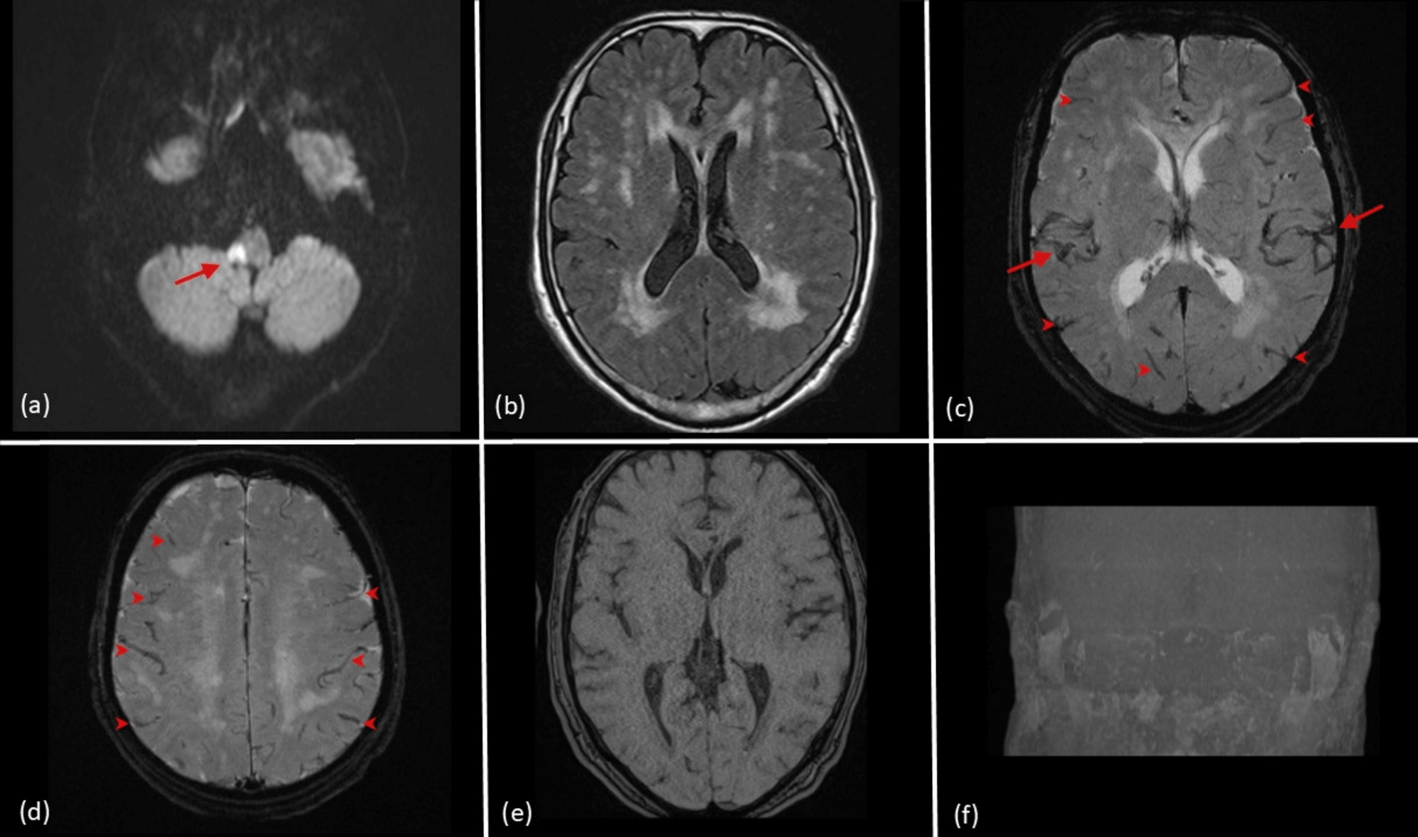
MRI findings during a follow-up scan. (a) Diffusion-weighted imaging shows a slight expansion of the ischemic area, with increased diffusion restriction in the right brainstem compared to the initial scan (arrow). (b) Fluid-attenuated inversion recovery image remains largely unchanged from the initial scan. (c) T2*-weighted imaging (T2*-WI) shows dilated cerebral arteries (arrows) and veins (arrow heads) as low signal intensity. (d) Another T2*-WI slice shows venous congestion as low signal intensity (arrow heads). (e) Magnetic resonance angiography (MRA) source image displays the absence of arterial blood flow signals, which was initially misinterpreted as a technical malfunction. (f)  Maximum intensity projection of the MRA confirms the complete loss of cerebral arterial flow, correlating with the patient’s cardiac arrest.
